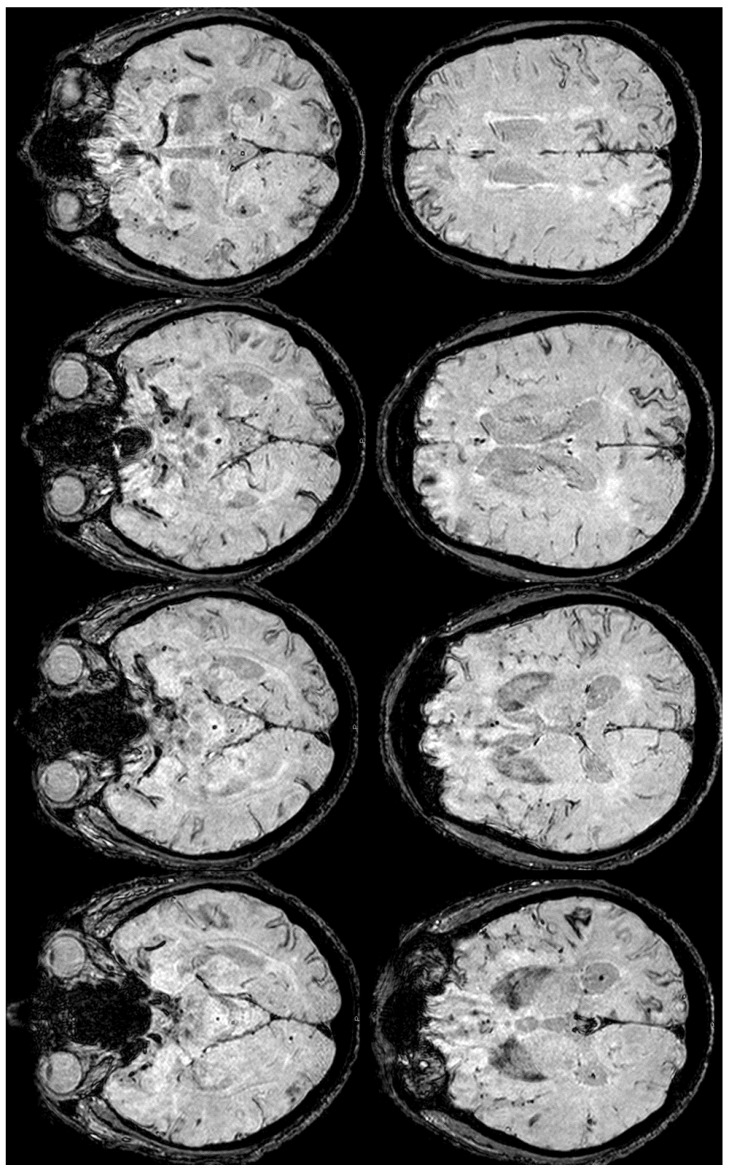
SWI-MRI in axial sections, showing an extensive, diffuse cSS, involving both occipital, temporal, and frontal lobes together with the left parietal lobe. In comparison with the previous MRI, an increased number of sulci were involved.
